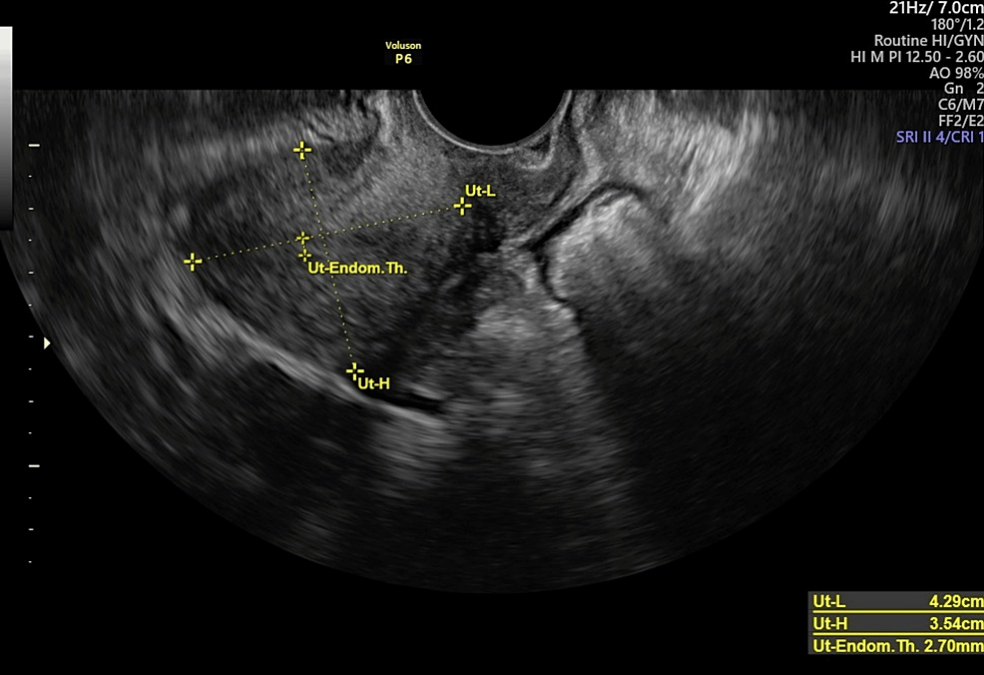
Ultrasound assessment of the uterine sizes and endometrial thickness before pregnancy
